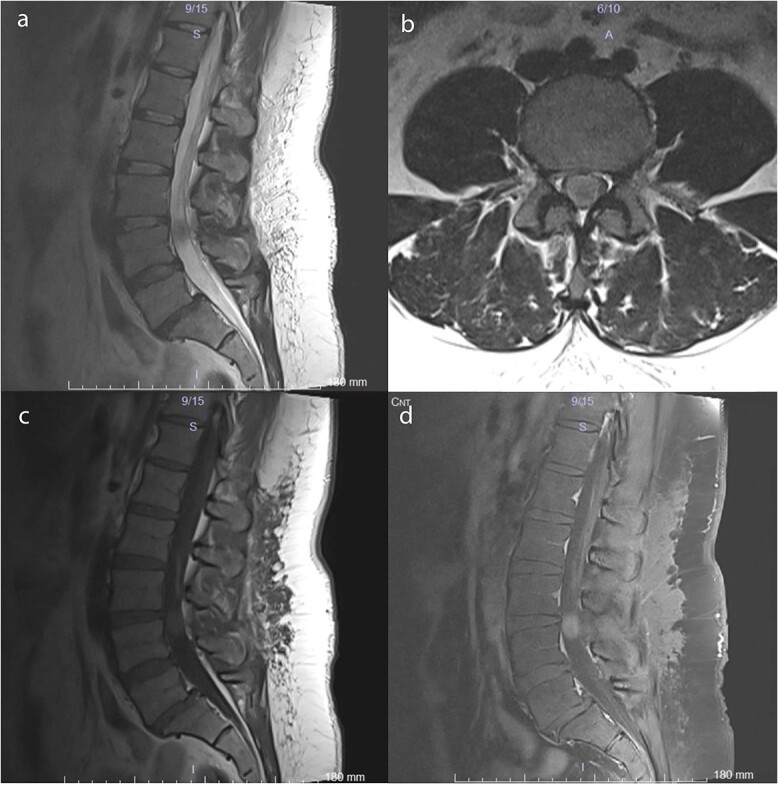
Preoperative MRI scans demonstrate a 17 × 12 × 15 mm intradural lesion posterior to the L3–4 disc and upper part of the L4 corpus. (a) The edge of the hypointense lesion is not so sharp in the midsagittal *T*_2_-weighted image. (b) In contrast to the sagittal *T*_2_-weighted image, the tumor appears to be hyperintense in the axial *T*_2_-weighted image. Because the nerve fibers are compressed by the tumor toward the dura CSF is not visible. (c) Midsagittal *T*_1_-weighted image shows a barely noticeable intradural tumor that is isointense with the vertebral body. (d) On midsagittal contrast-enhanced *T*_1_-weighted image, the tumor was homogeneously enhanced and became prominent.
